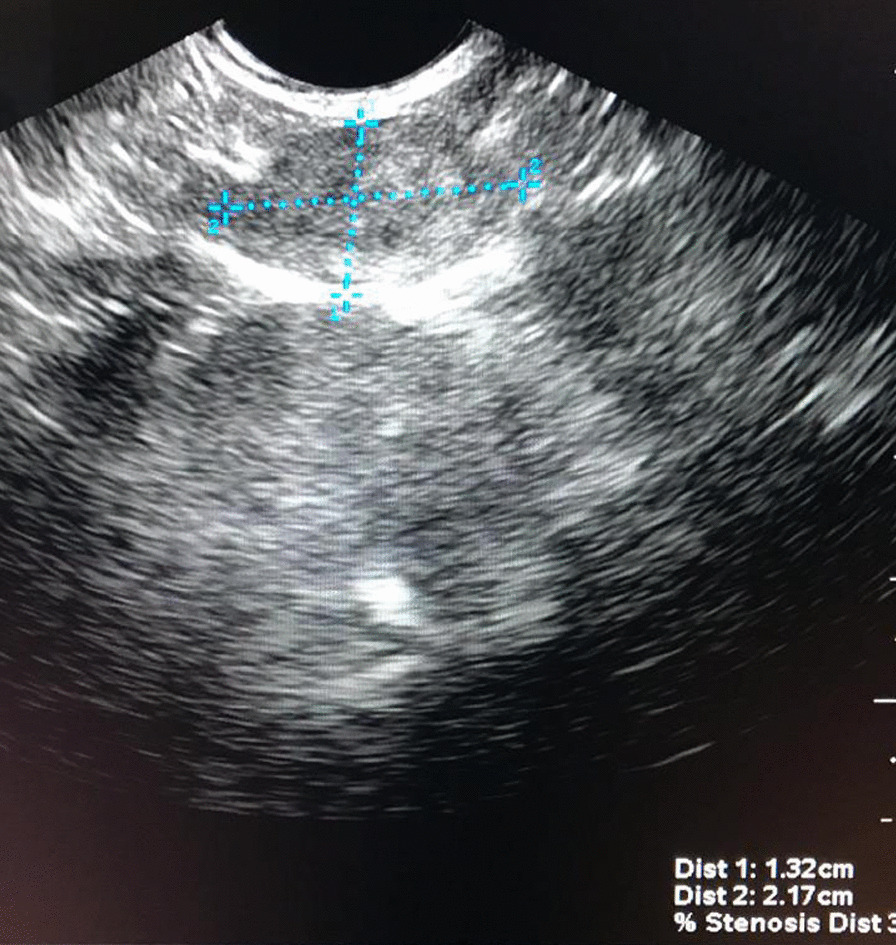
Endoscopic ultrasound of the patient showing a pancreatic nodule
